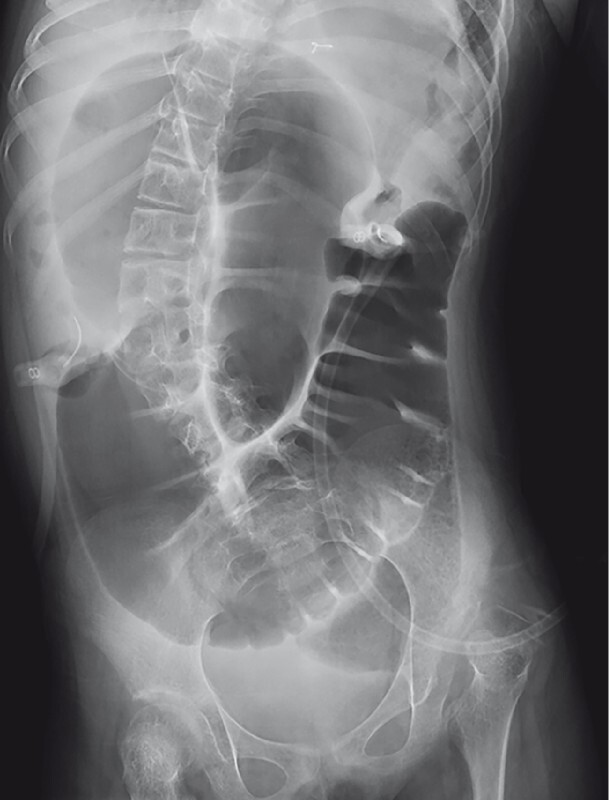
Abdominal radiograph shows a huge dilated colon.
